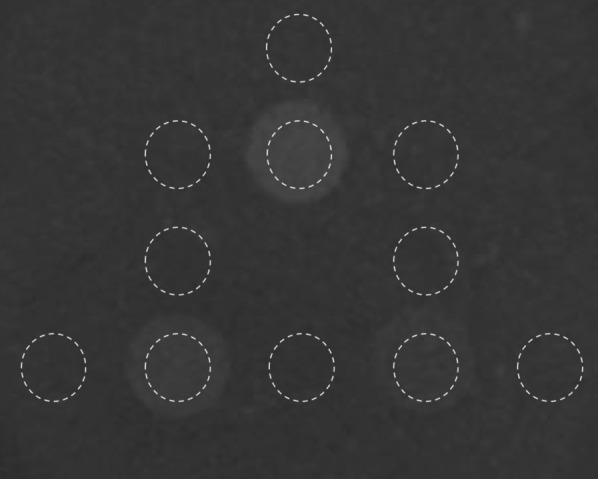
The layout of background ROIs for CT image quality analysis
